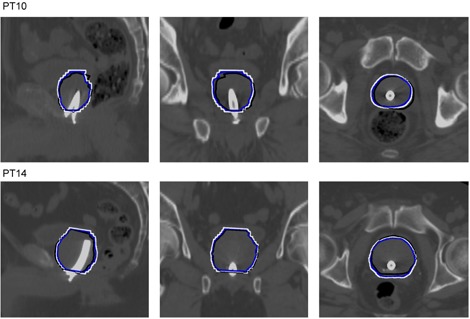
The order is sagittal, coronal, and axial views of the overlap of the manual prostate delineation performed in the original MR image and then aligned to the CT based on the manual registration (black), the automatic registration of the CTV (blue), and the automatic registration of the extended CTV (white). The delineations are superimposed on the CT images.
